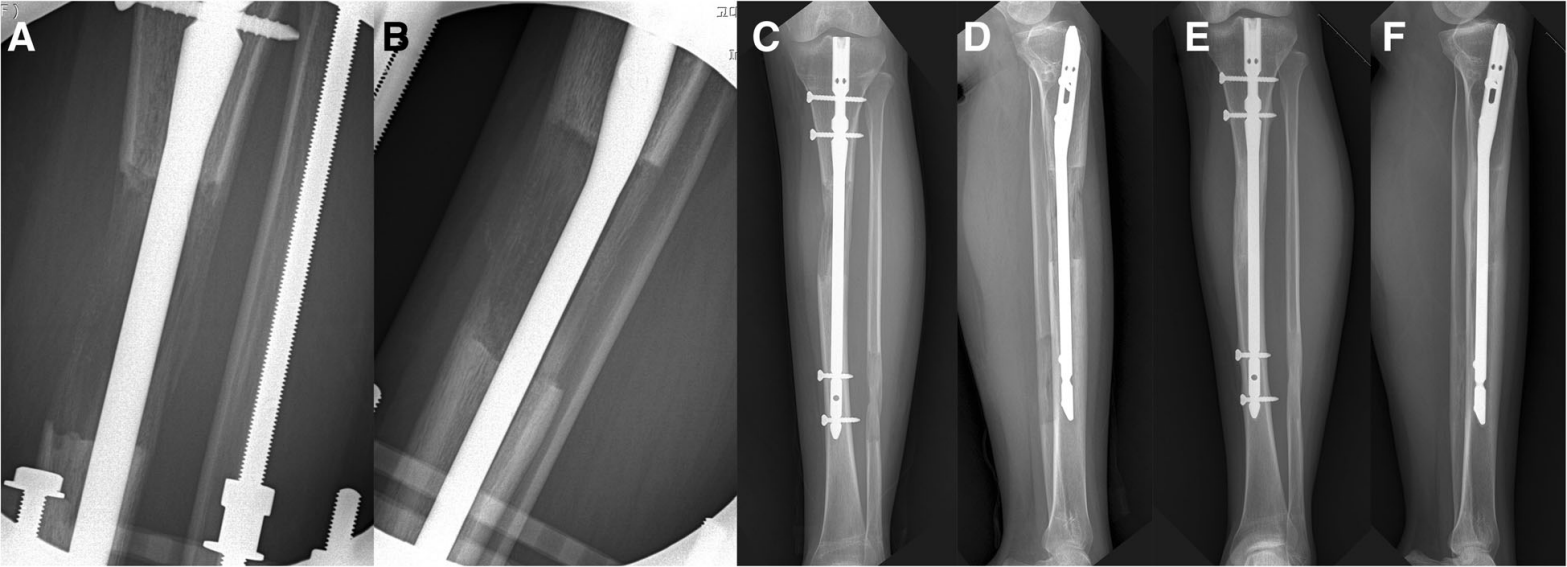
Sequential radiographic images of the left tibia in a 22-year-old female patient who received low-intensity pulsed ultrasound (LIPUS) after 7.3 cm of tibial lengthening. At the end of the distraction phase, the anteroposterior (AP) (a) and lateral (b) images show a callus with a cylindrical shape and intermediate density. At the time of external fixator removal, the AP (c) and lateral (d) images show progression of callus consolidation with a cylindrical shape and normal density. The external fixation index of the patient was 29.6 days/cm. At 9 months postoperation, the AP (e) and lateral (f) images show full corticalization of the regenerated callus
